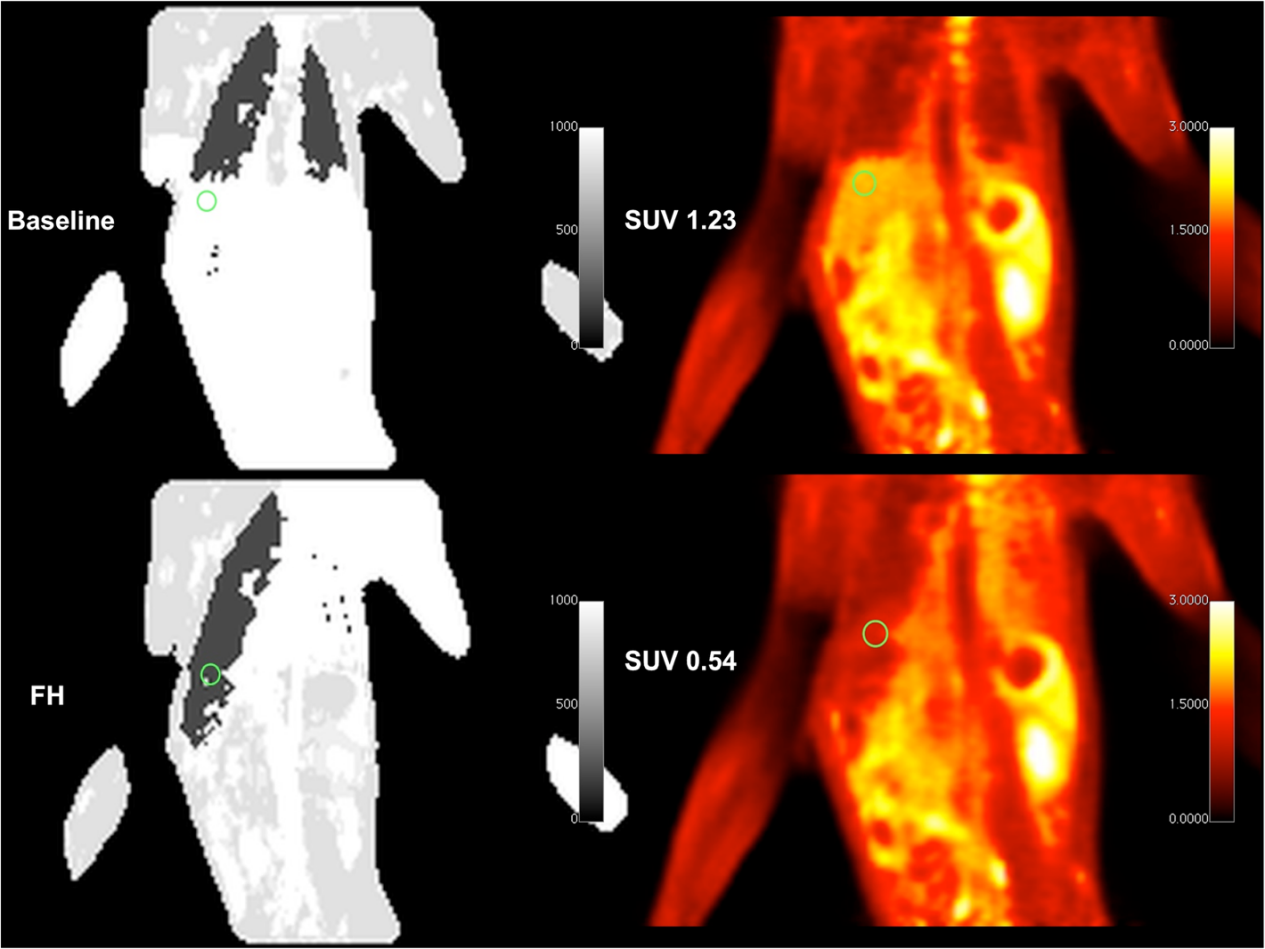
Effects of ferumoxytol on measured SUV. Coronal mu-maps (left column) and PET SUV images (right column) showing a typical ROI in the liver region. The top row is data obtained at 5 min after 10 mg/kg ferumoxytol administration (SUV 1.23); the bottom row is data obtained 57 min after ferumoxytol administration (SUV 0.535). PET raw data is identical in both instances; however, attenuation correction has been performed with the mu-map on the left of each PET dataset. Please note the significant liver area misclassification at the 57-min time point (signal drop due to high liver iron content).
